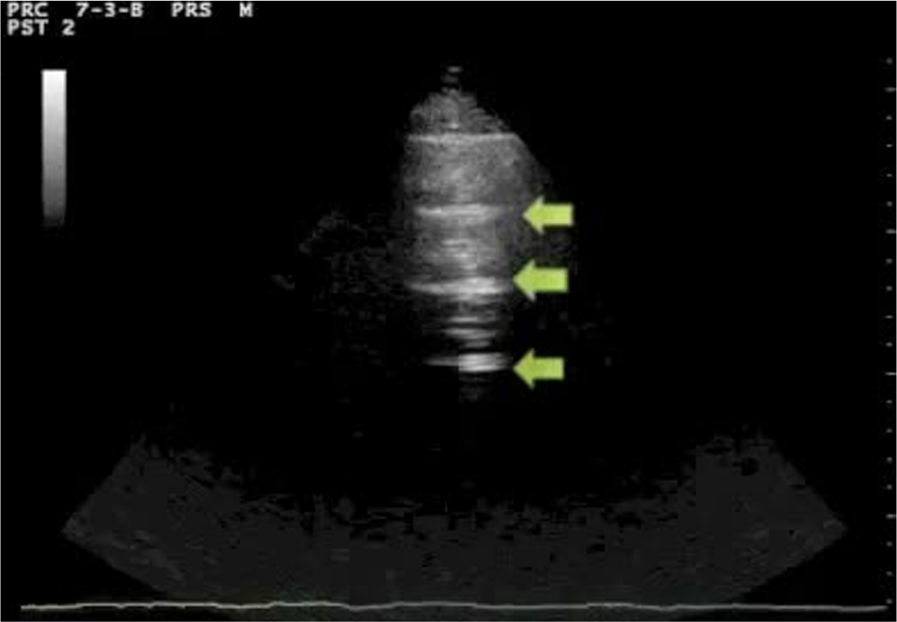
Sonographic appearance of an aerated lung scan. Arrows indicate A-lines. Above A-lines the pleural line is visible with its horizontal movement, the lung sliding.
